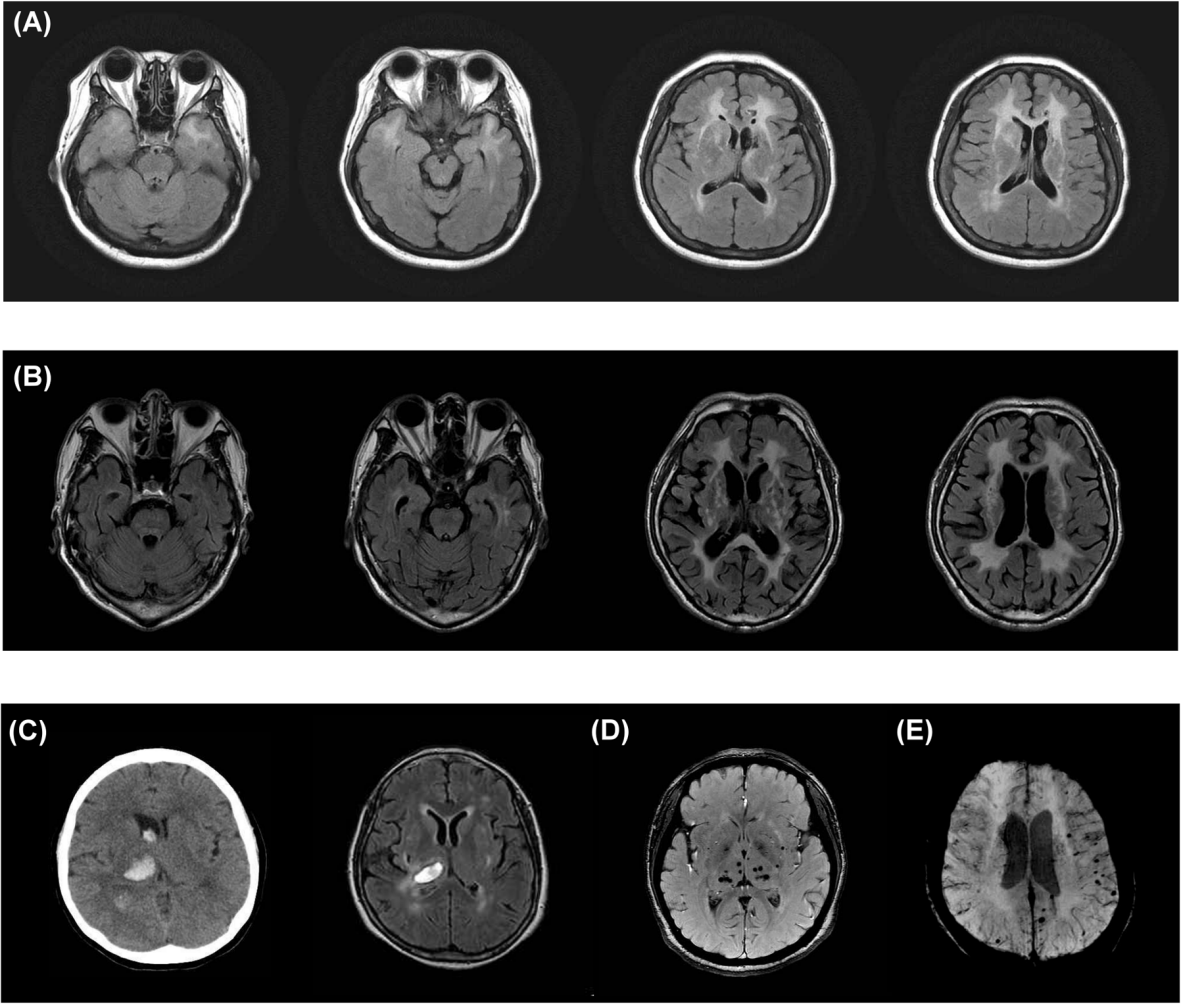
Representative neuroimaging of CADASIL patients. (A) Fluid-attenuated inversion recovery (FLAIR) images from a 54-year-old patient with *NOTCH3* R110C mutation showing diffuse white matter lesions (WMLs) with significant involvement of external capsules and anterior temporal pole. (B) FLAIR images from a 62-year-old patient with *NOTCH3* R544C mutation showing diffuse WMLs without significant involvement of anterior temporal pole. (C) Brain computer tomography and T2 weighted images from a 55-year-old patient with *NOTCH3* R544C mutation showing diffuse WMLs and an intracerebral hemorrhage (ICH) in the right thalamus on the second and eighteenth day, respectively, after the occurrence of ICH. (D) Susceptibility-weighted angiography (SWAN) images from a 48-year-old patient with *NOTCH3* R427C mutation showing multiple cerebral microbleeds in the thalami and basal ganglia. (E) SWAN images from a 53-year-old patient with *NOTCH3* R544C showing multiple cerebral microbleeds in the subcortical regions.
